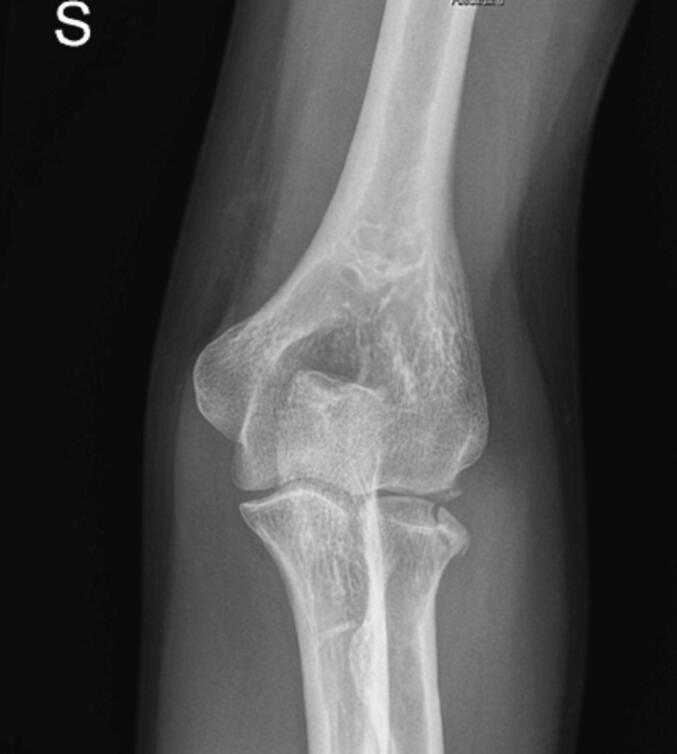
Xray elbow after 1 month.
